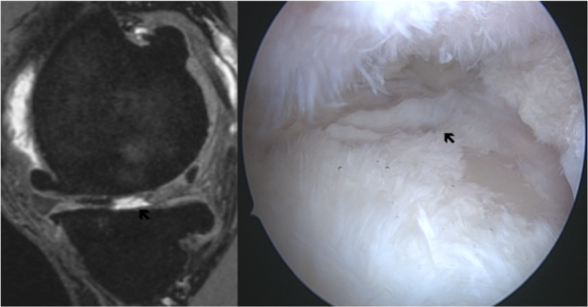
MRI and arthroscopic image of a grade IV lesion in a 42-year-old patient completely depleted of cartilage in the weight bearing area
